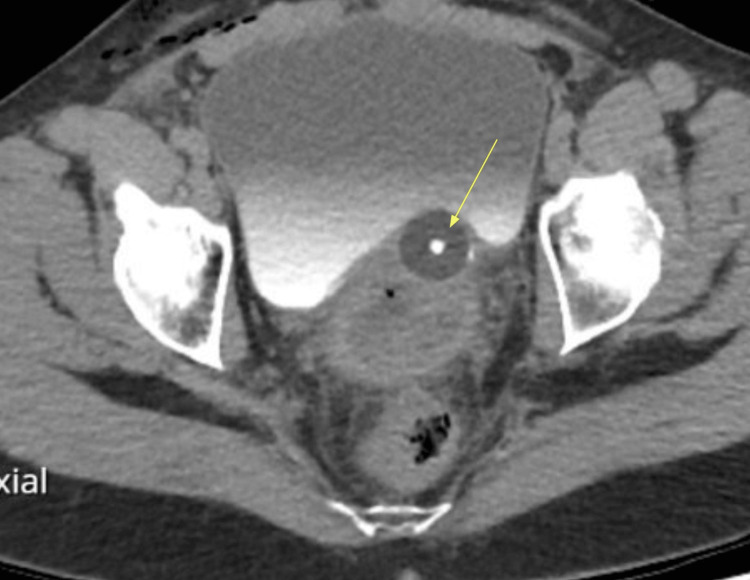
Axial CT intravenous pyelogram demonstrating urinary catheter malposition outside of the urinary bladder.
